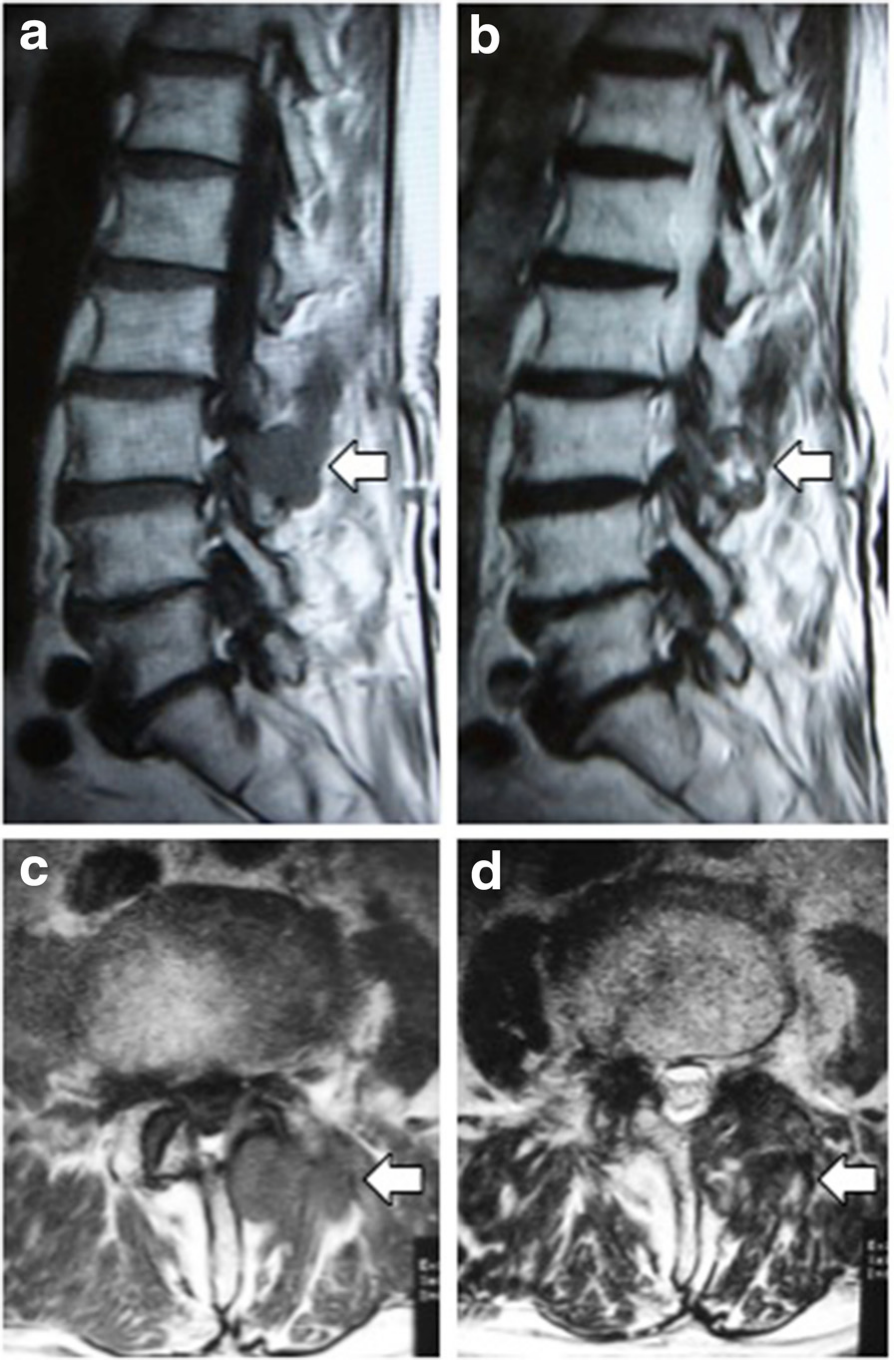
Magnetic resonance imaging sagittal and axial images. A soft tissue mass (open arrow) at the L3 to 4 level is hypointense compared with the spinal cord on the T1-weighted image (a, c), and heterogeneous signal intensity is seen on the T2-weighted image (b, d).
